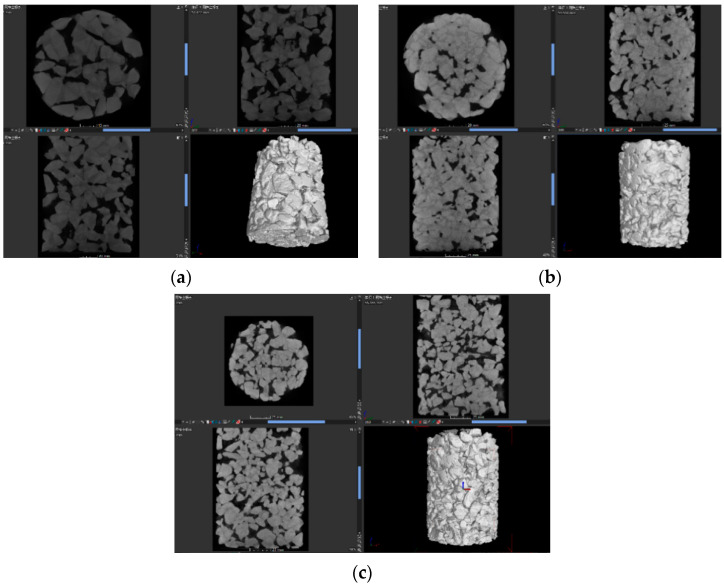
3D CT reconstruction of macropore structure in EPC: (a) *P* = 20%, aggregate 5–25 mm; (b) *P* = 30%, aggregate 5–25 mm; (c) *P* = 30%, aggregate 16–25 mm [20].
